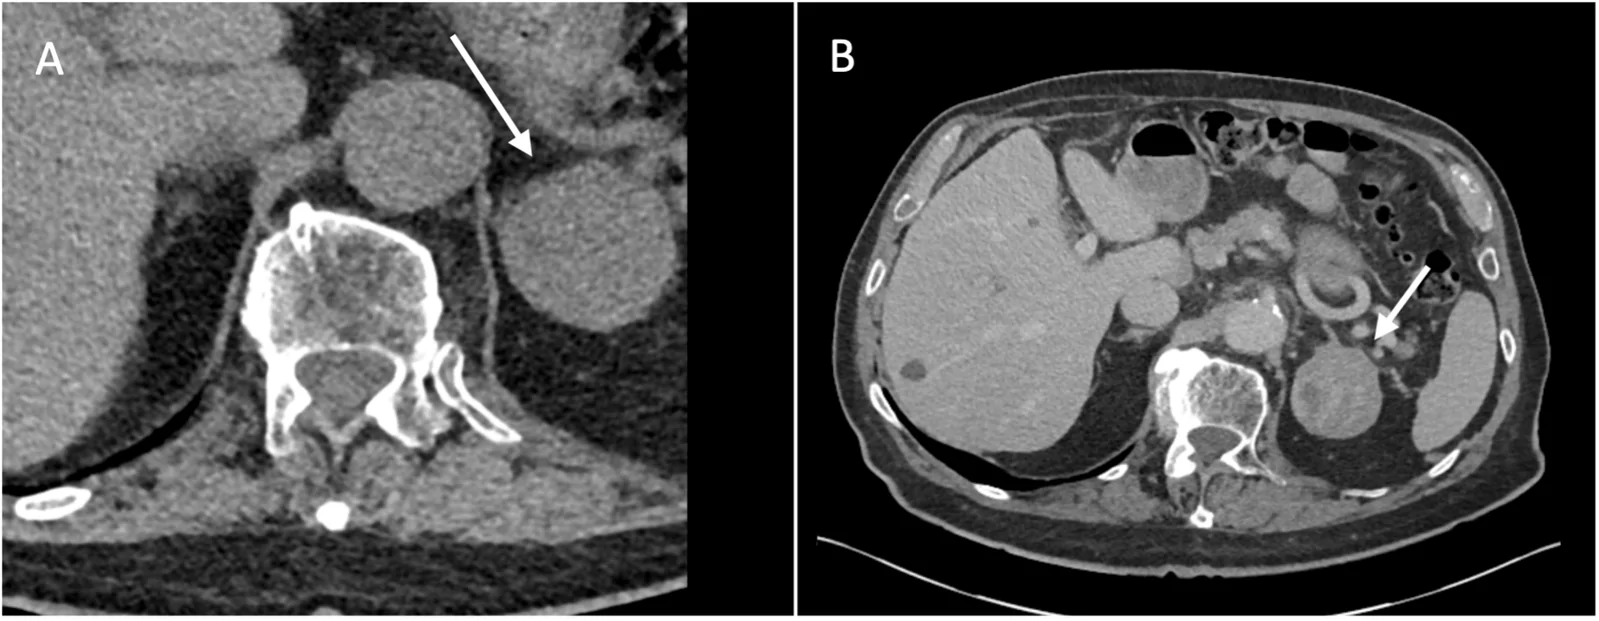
Tumor progression of the adrenal mass in three months (approx. 6mm). (A) Initial CT scan of the lumbar spine, (B) Follow-up CT scan of the abdomen (absolute washout 45%).
